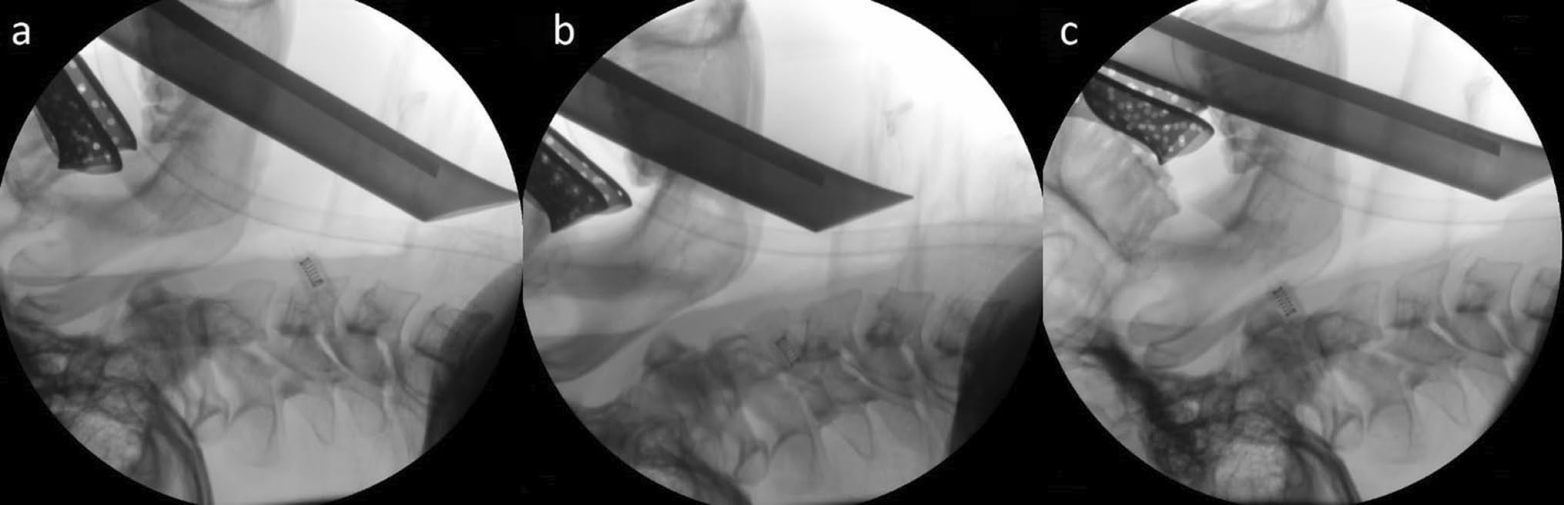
Intra-operative lateral X-rays of an informed anaesthetized patient, his head and neck were put in the three positions with the laryngoscope blade and the intubation tube inside
